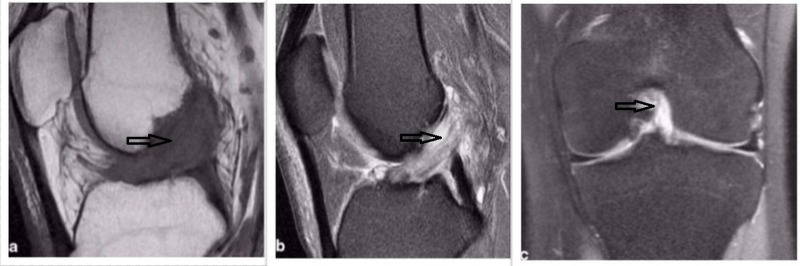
MRI showing ACL mucoid cyst a) T1-weighted sagittal view; b) T2- weighted sagittal view; c) T2- weighed coronal view MRI: magnetic resonance imaging; ACL: anterior cruciate ligament
